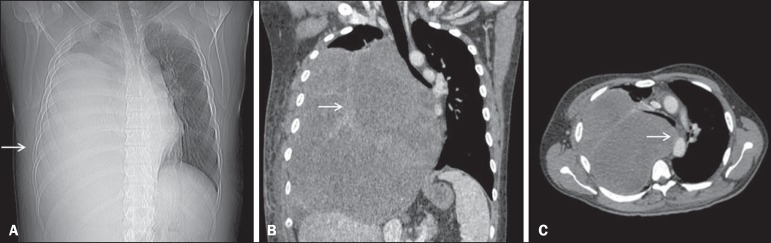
CT scan showing a primary sarcoma in the right hemithorax. A: CT
